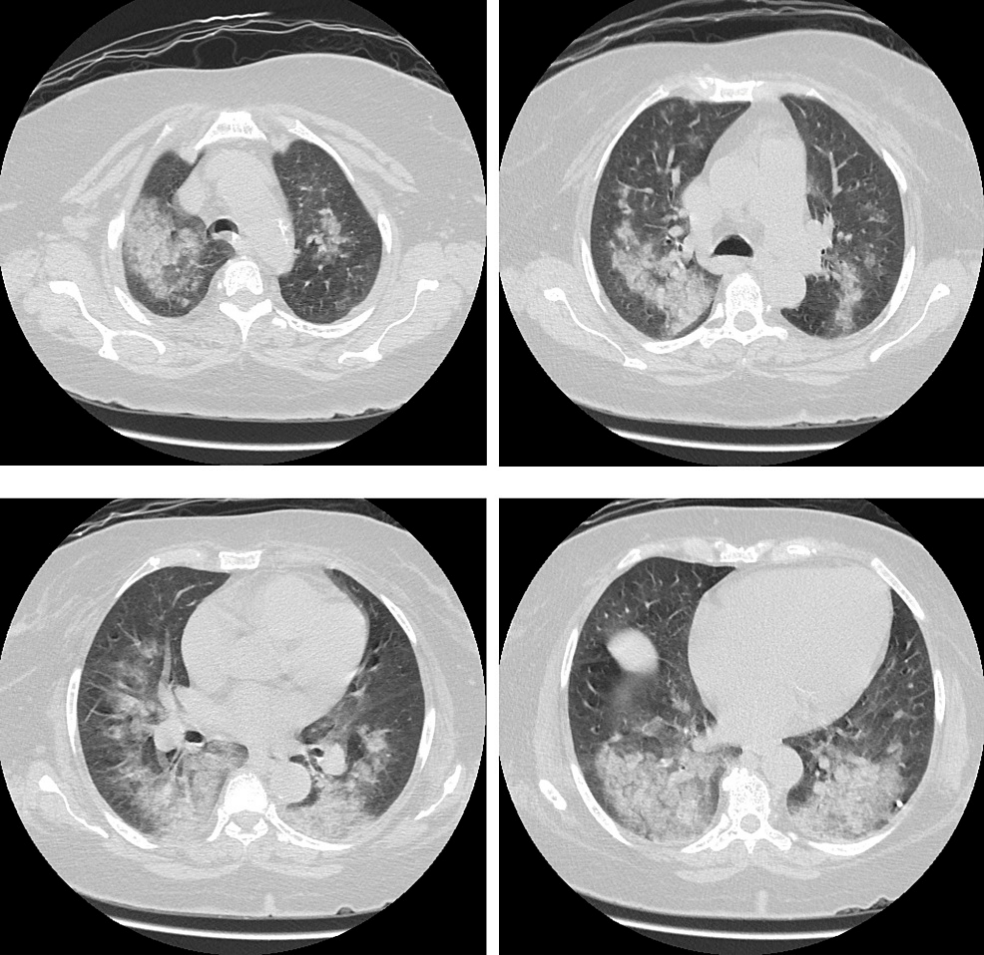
CT scan showing bilateral airspace opacity suggesting hemorrhage CT; computed tomography
